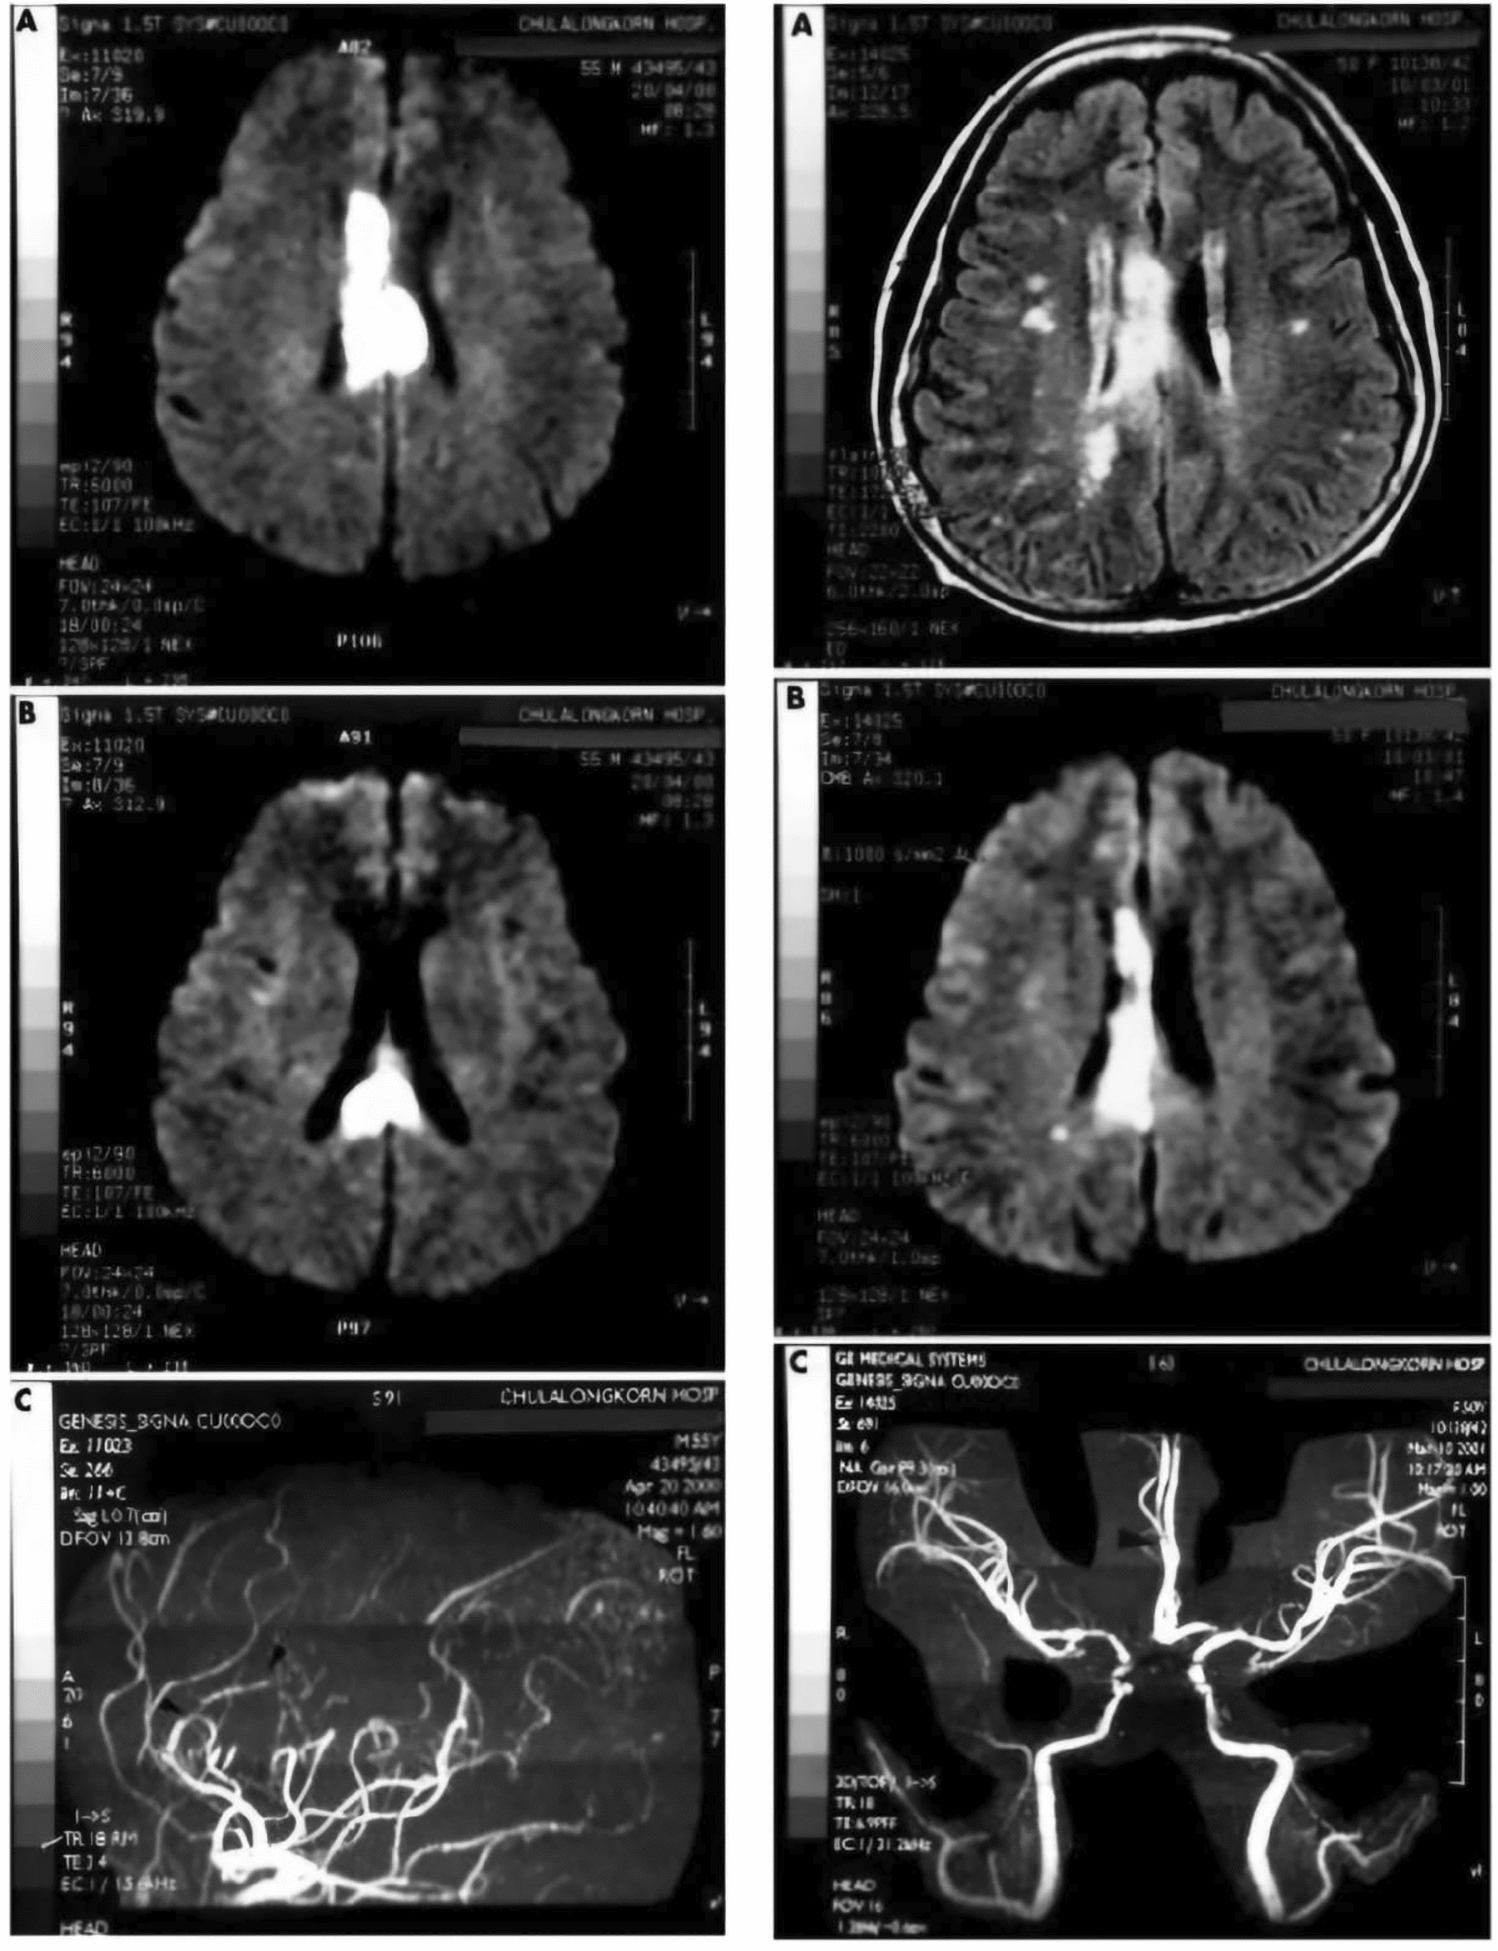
FLAIR/DWI showing Corpus callosum infarction (A-B) and magnetic resonance angiography showing pericallosal artery narrowing indicated by arrow (C); FLAIR: Fluid Attenuated Inversion Recovery, DWI: Diffusion-weighted imaging. [47] Copyright © 2001 N C Suwanwela, N Leelacheavasit
